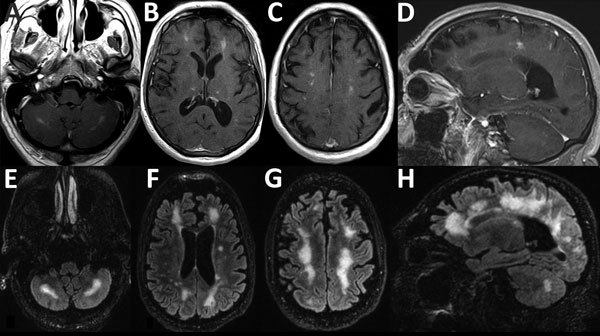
Magnetic resonance imaging scans showing brain abnormalities in a previously healthy adult with *Baylisascaris* meningoencephalitis, California, USA. A–D) Postgadolinium contrast T1 images obtained 4 weeks after symptom onset. A–C) Axial images, moving inferiorly to superiorly, demonstrating nodular bilateral enhancement within the cerebellar hemispheres, thalami, and subcortical white matter. D) Sagittal image further demonstrates multifocal areas of enhancement in cerebral hemispheres. Additional, mild T2 abnormalities (not shown) were present at the same time. E–H) T2/FLAIR (fluid attenuation inversion recovery) images obtained 6 weeks after symptom onset. E–G) Axial images, moving inferiorly to superiorly, demonstrating patchy and confluent hyperintense lesions throughout the supratentorial white matter and cerebellum. H) Sagittal image further demonstrates the high degree of white matter abnormality, which was not present on the earlier imaging. Postcontrast enhancement on T1 imaging (not shown) had nearly resolved at this time.
